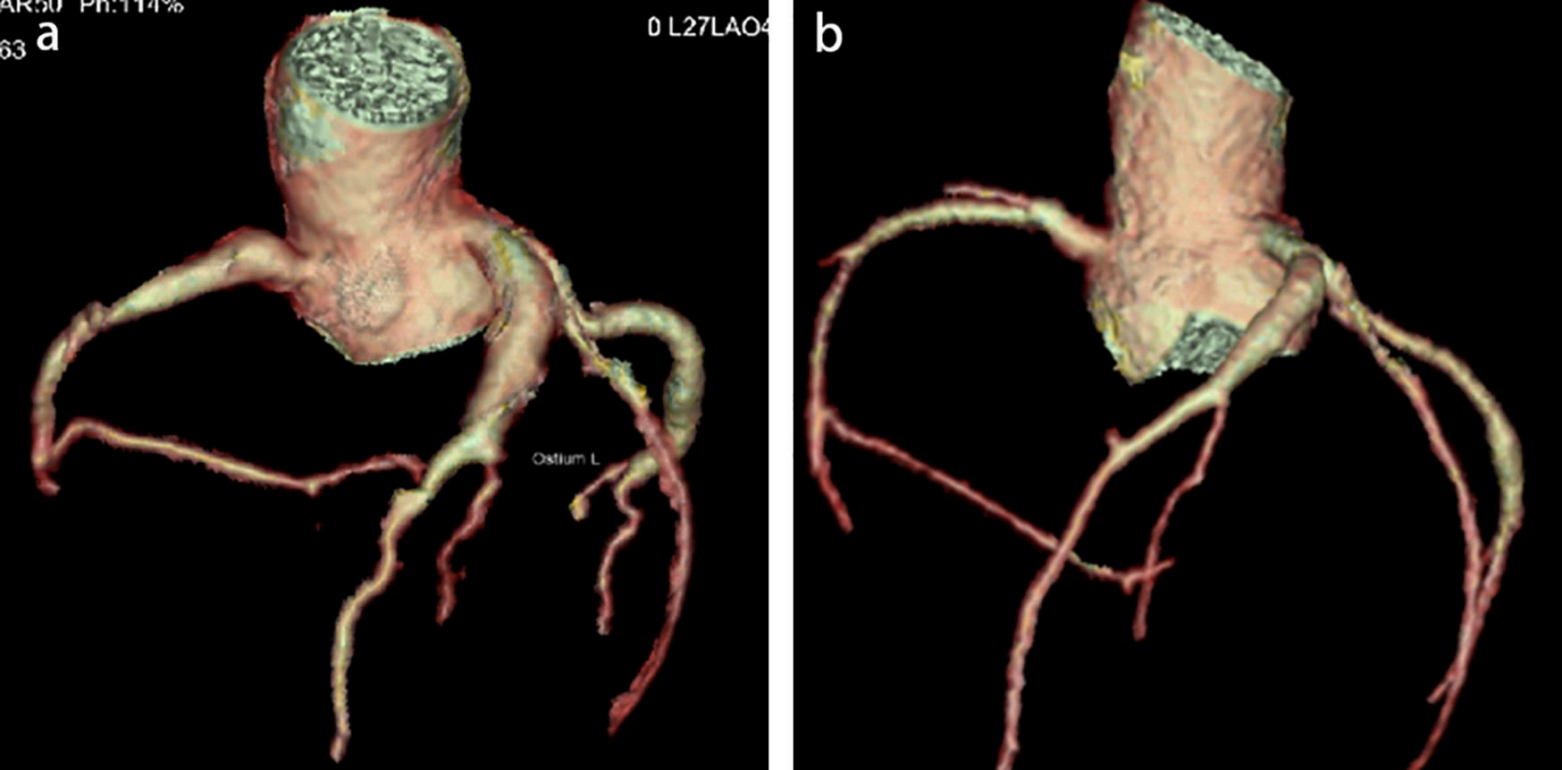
Coronary artery computed tomography angiography (CTA, 3 months after the onset of illness). CTA before discharge (a) shows long segment aneurysms in RCA, LAD, and LCX, respectively, with a maximum diameter of 6.8 mm in LAD. CTA at 3 months follow-up (b) shows significant improvement in every three branches, with a maximum diameter of 4.8 mm in LAD.
